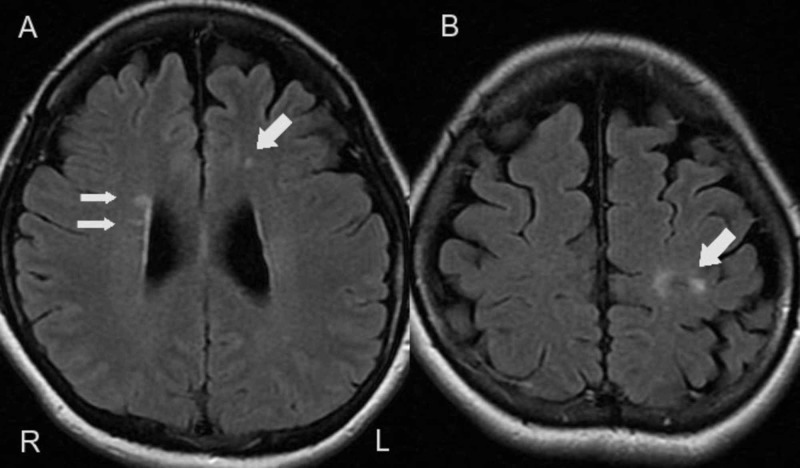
Brain MRI findings. In the cerebrum, fluid attenuation inversion recovery axial imaging showed that multiple white matter lesions were presented around the lateral ventricles (A) and juxtacortical region (B).
